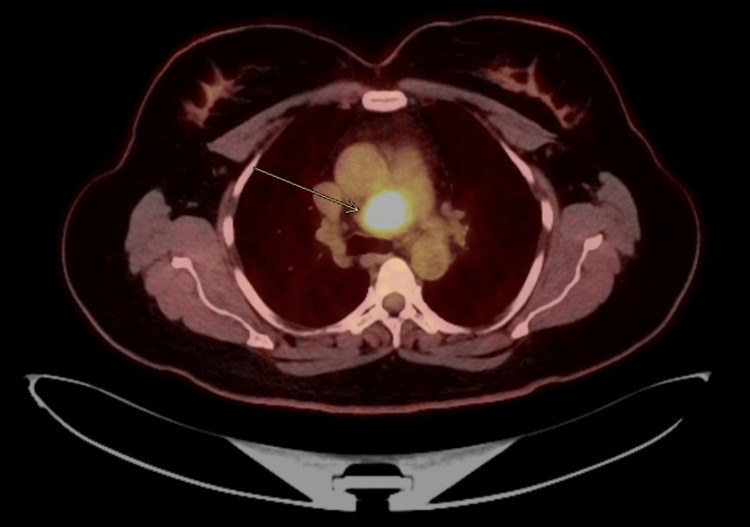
PET-CT scan axial view demonstrating a moderately fluorodeoxyglucose (FDG) avid lower paratracheal mass
